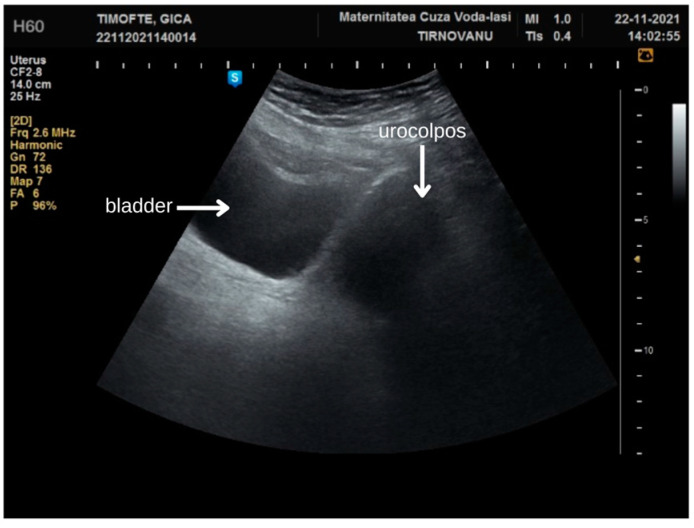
Ultrasound at first admission for labial fusion.
